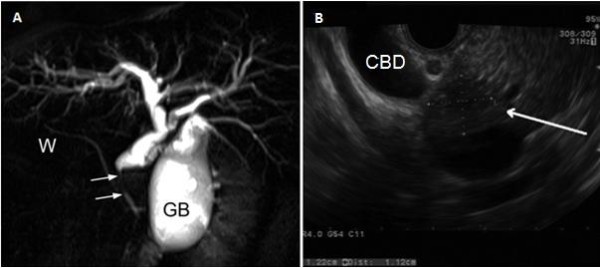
A: Magnetic resonance cholangiopancreatography shows a tapered stricture of the common bile duct (arrows) with upstream dilation of the bile ducts.B: Endoscopic ultrasonography shows an hypoechoic nodule (arrow) within the head of the pancreas and close to the dilated common bile duct. GB: gallbladder; W: Wirsung duct; CBD: common bile duct.
